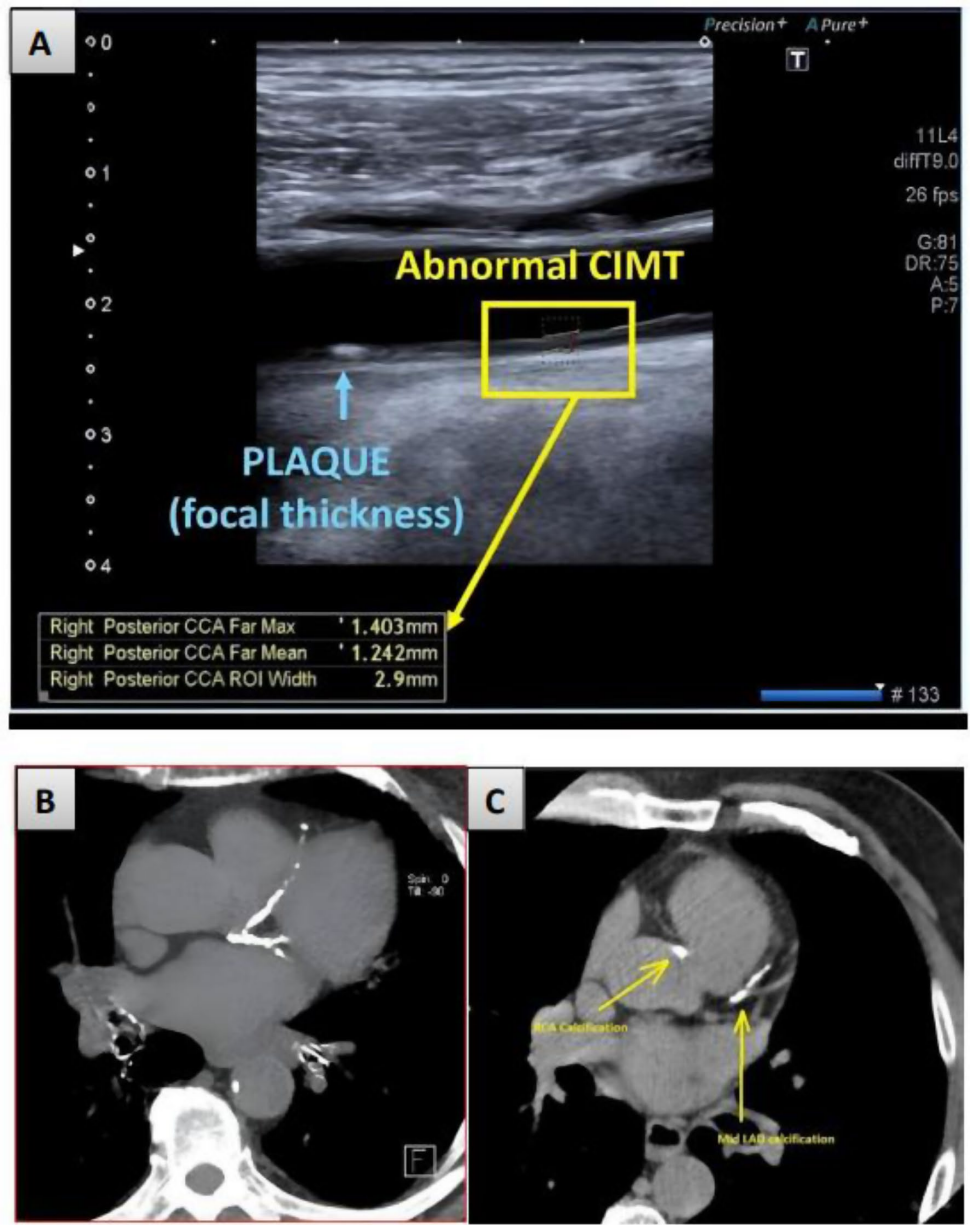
Carotid duplex and cardiac CT scan in the studied patients: (A) Carotid duplex showing increased carotid intima media thickness (CIMT) and carotid atheromatous plaque; (B) Cardiac CT demonstrating heavily calcified coronary vessels with a score over 2000; (C) Cardiac CT demonstrating coronary calcification in left anterior descending coronary artery (LAD) and right coronary artery (RCA)
